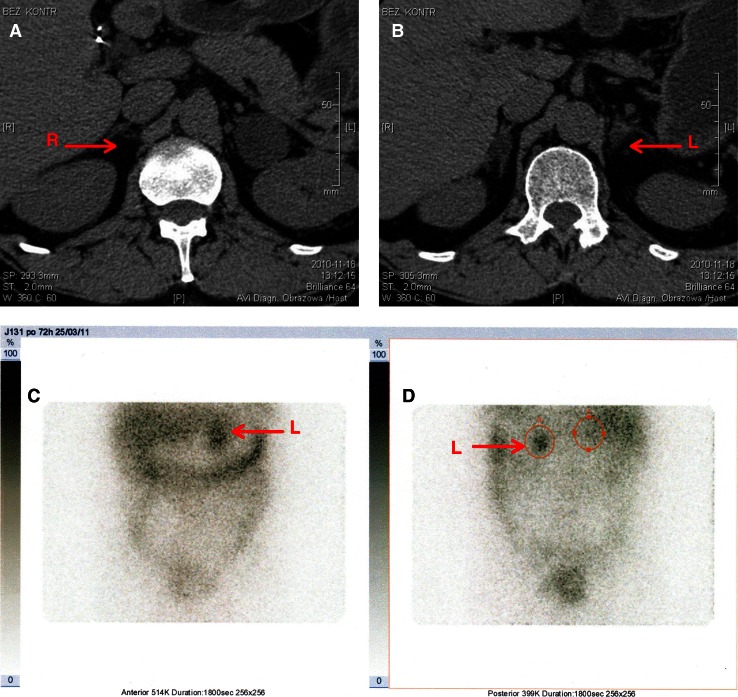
Predominant radiotracer accumulation (85%) in the left adrenal gland (with the bigger tumor). a, b CT (the arrows point to the both tumors); c anterior planar view of scintigraphic study; d posterior planar view.
